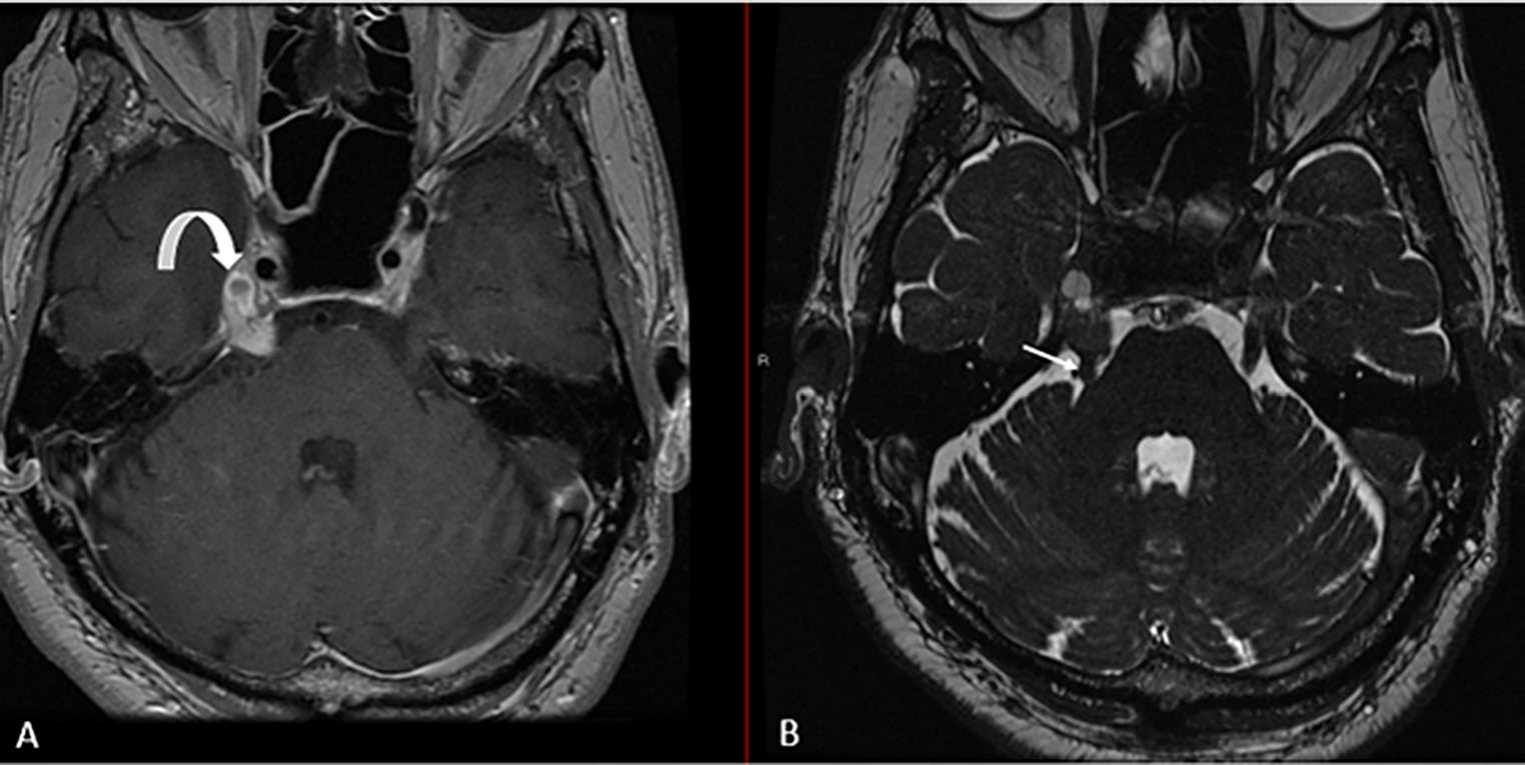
MRI brain axial T1 post-contrast (A) and axial T2 (B) show a heterogeneously enhancing mass involving the distal cisternal segment of the right trigeminal nerve (white arrow) and expanding the right Meckel’s cave; a non-enhancing cystic component is seen in Meckel’s cave (curved arrow)
